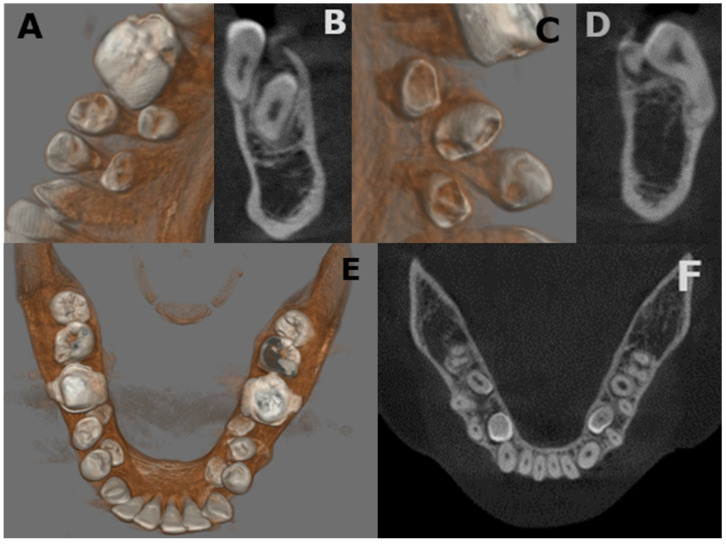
(A–F) CBCT findings of a 21-year-old: (A–D) rendering and axial slices of supplementary inferior premolars; (E) rendering of mandibular view; (F) coronal slices. Lingual position of the lower impact teeth in areas 4.4–4.5 and 3.4–3.5.
